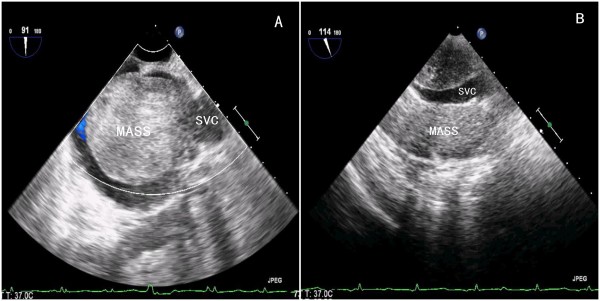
Transesophageal echocardiography demonstrated a giant mass arising from the SVC. A) Transesophageal echocardiography revealed a giant mass arising from the anterior wall of the superior vena cava (SVC); B) The cavity of the SVC was occupied by the mass.
